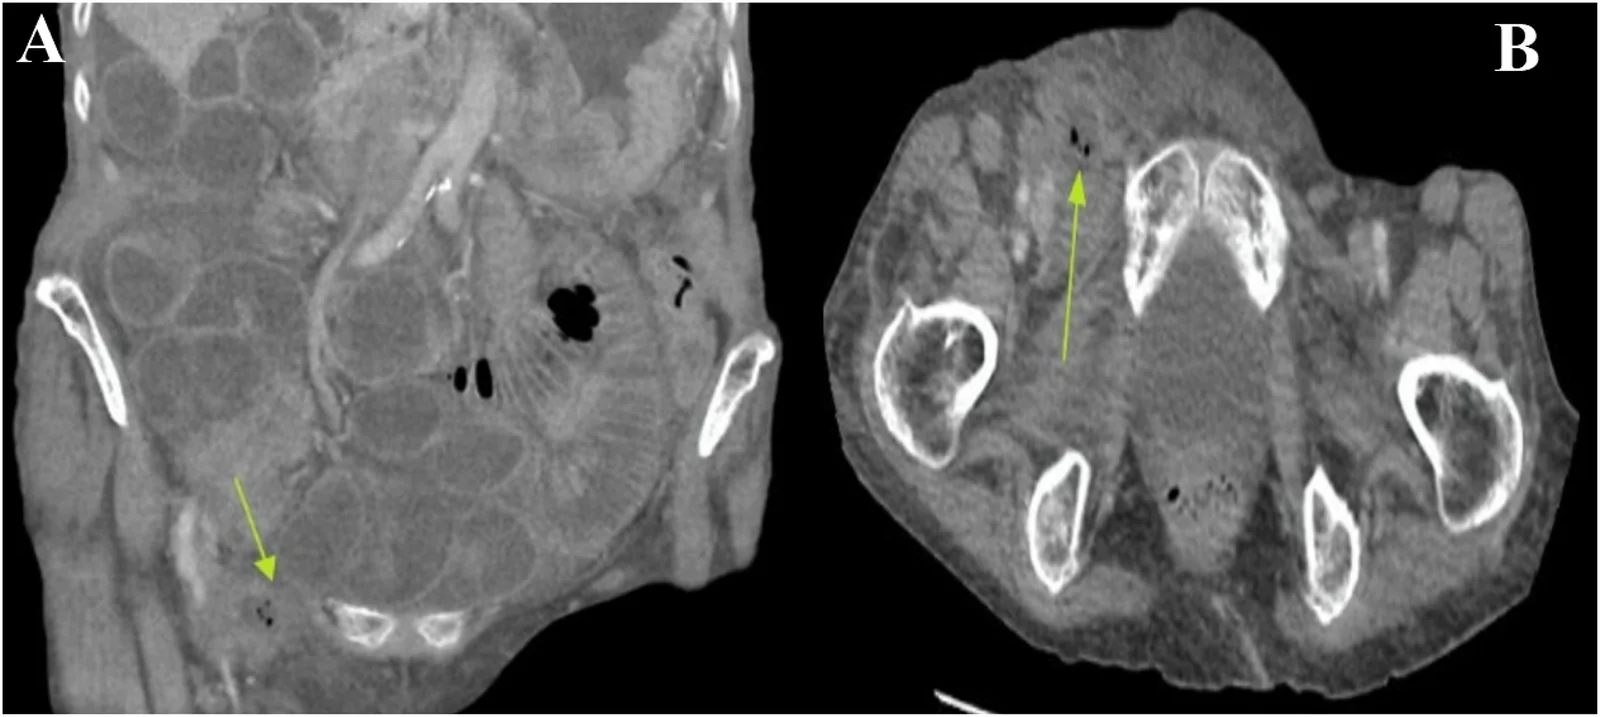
Abdominopelvic CT. (A) Coronal reconstruction: dilated small bowel loops with air-fluid levels (intestinal obstruction); the arrow indicates the herniated content within the right femoral defect. (B) Axial section at the level of the pubic symphysis: the arrow identifies the herniated content within the right femoral canal, medial to the femoral vessels, with a focus of gas, corresponding to De Garengeot hernia.
